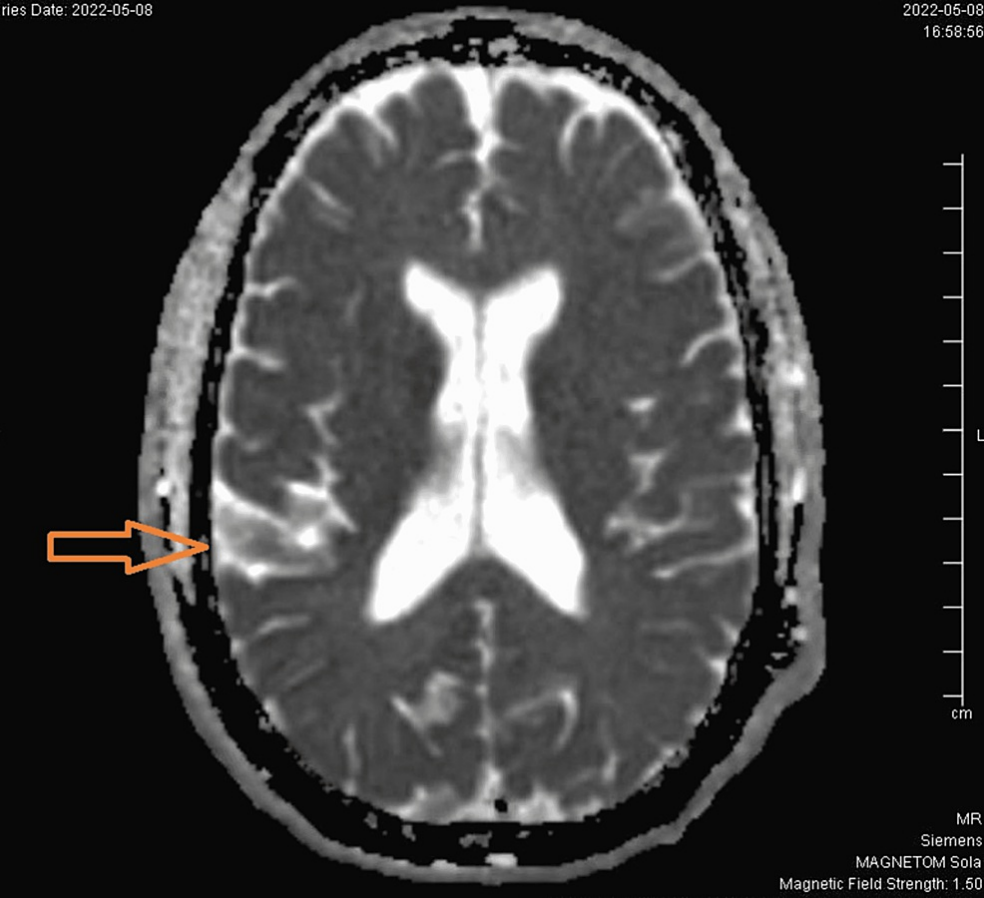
Temporoparietal ribboning of the gyri seen in the right side DWI image DWI: diffusion-weighted imaging
